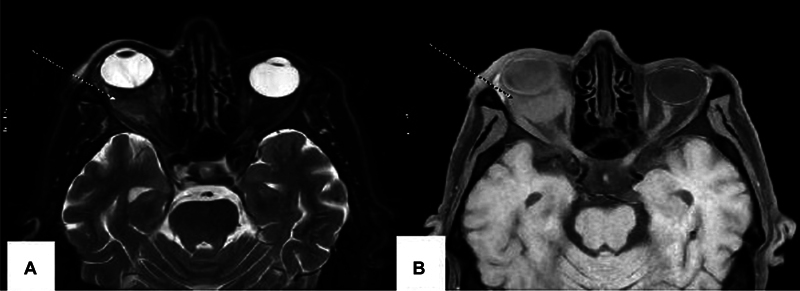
Magnetic resonance imaging (MRI) of the orbital region showing a 30 × 30 × 22 mm right retro-orbital mass isointense on T1 (
